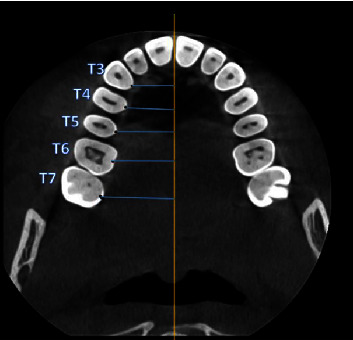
CBCT-axial view shows the definition of measurement points. Each point was marked from the midpalatal of the cementoenamel junction (CEJ) to the middle palatine suture (blue line) and the yellow line resembles the middle palatine suture.
